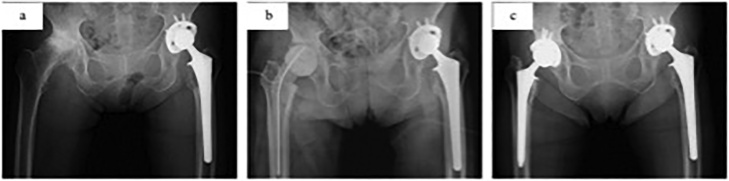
Anterior-posterior plain radiograph of the hip joint. (a) Plain radiograph before 1st-stage operation. (b) Plain radiograph after 1st-stage operation. (c) Plain radiograph at final follow-up.
